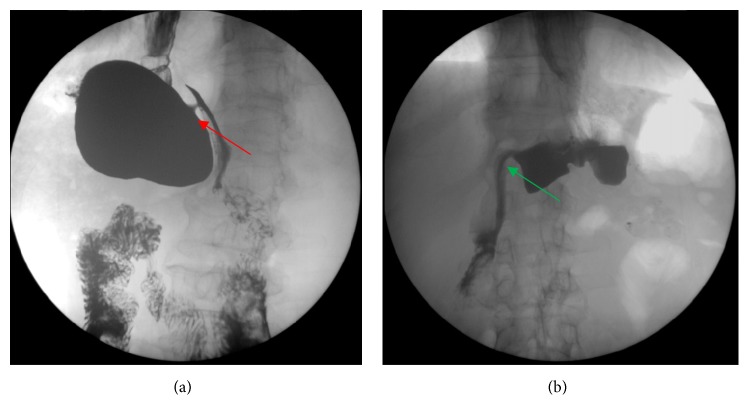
(a) Upper gastrointestinal radiography before G-POEM showing a narrow pyloric canal (shown with a red arrow) in the patient post-subtotal gastrectomy and (b) upper gastrointestinal radiography showing contrast passing freely through the pylorus after G-POEM (shown with a green arrow).
